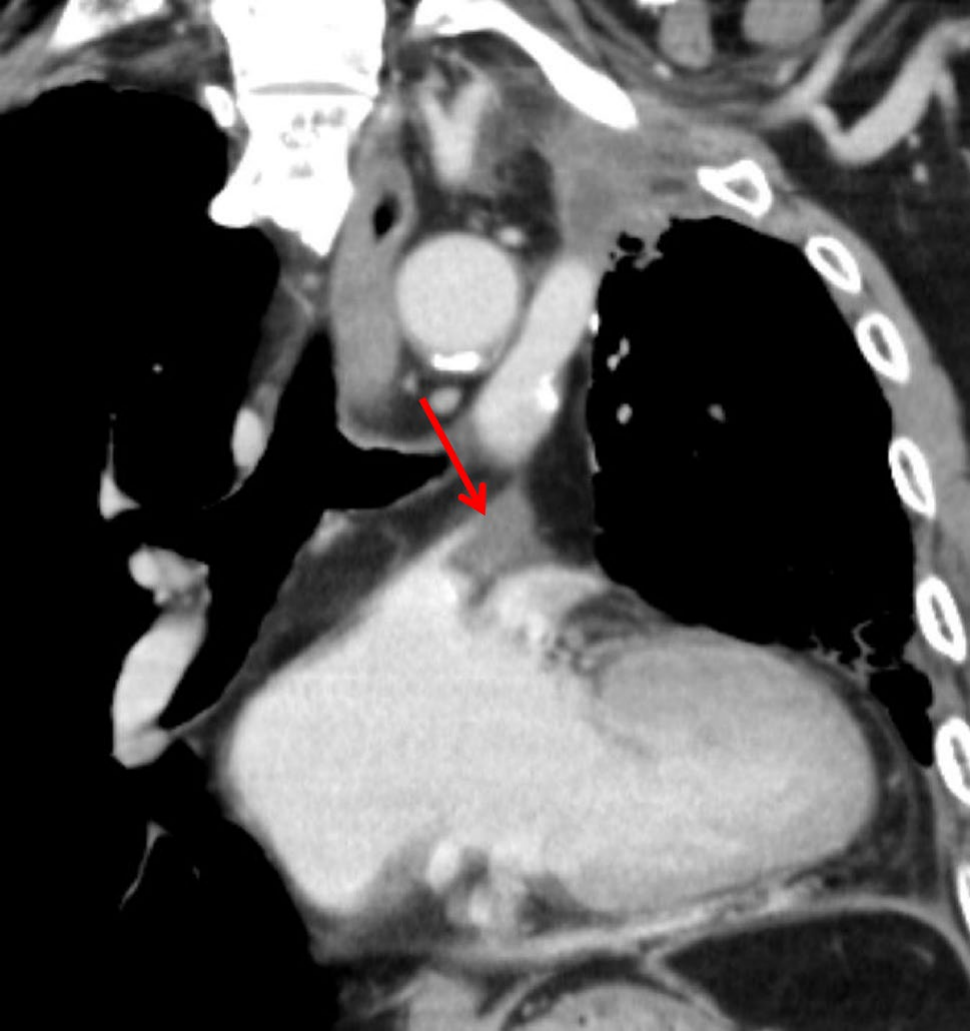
Thrombosis in the pulmonary vein stump. Eight months after left upper lobectomy, contrast-enhanced coronal CT shows a relatively elongated left superior pulmonary vein stump with a filling defect, consistent with thrombus formation (arrow)
